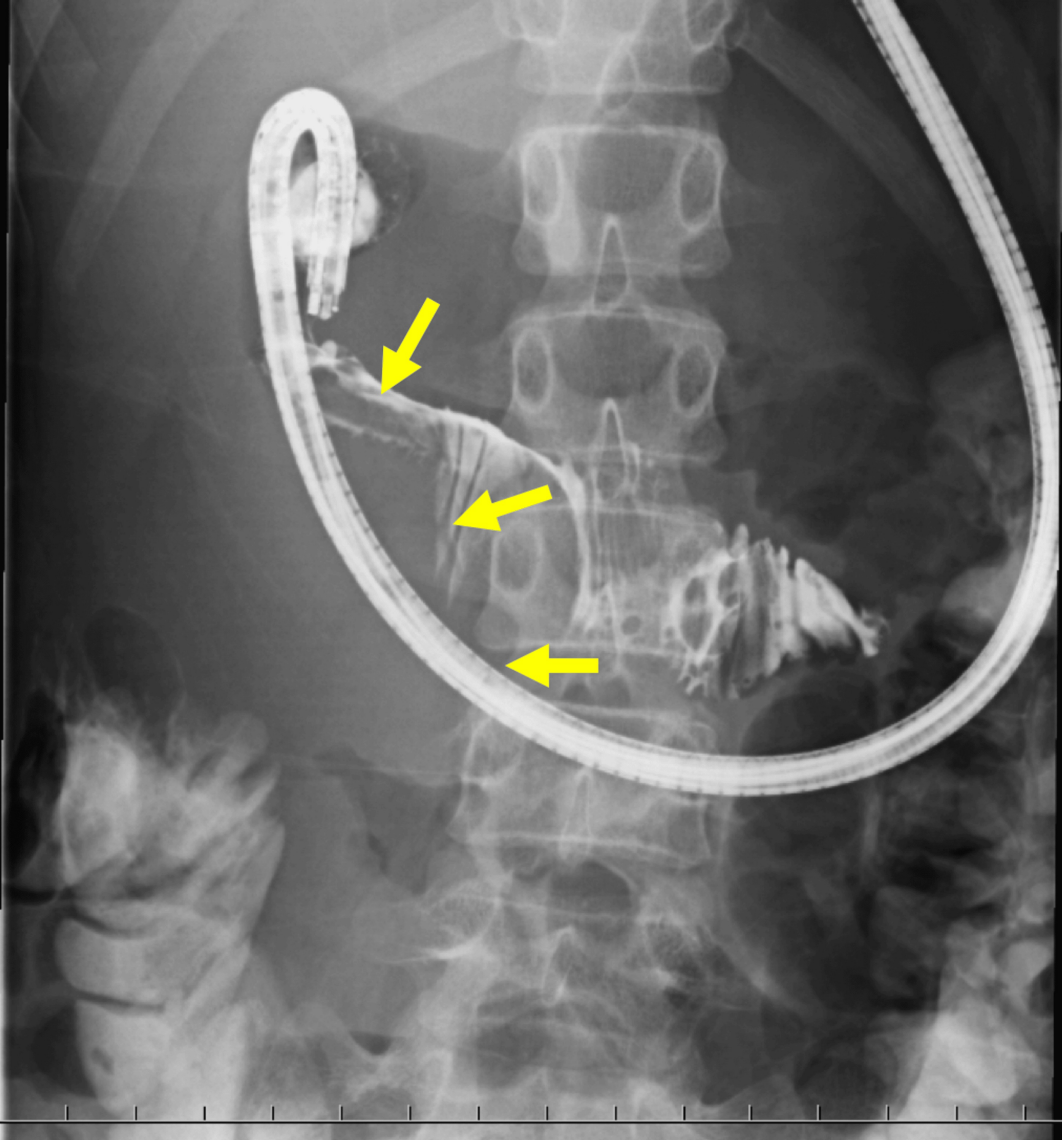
Esophagogastroduodenoscopy and contrast radiography. The intramural duodenal hematoma (arrow) compressed the descending portion of the duodenum.
